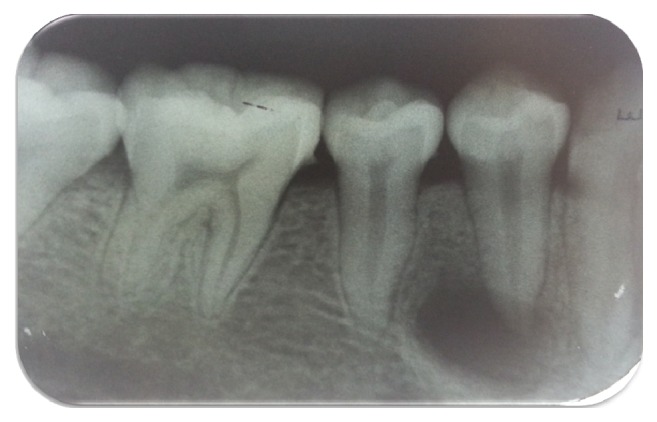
Diagnostic IOPAR, periapical radiolucency with respect to 44.
